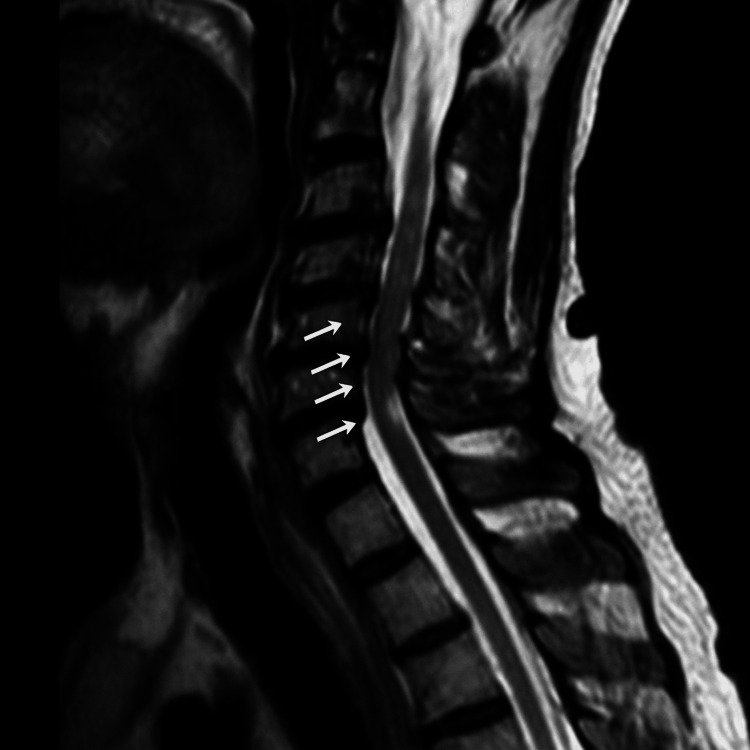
MRI spine showing cervical spondylosis with patchy T2 hyperintensities (arrows).
